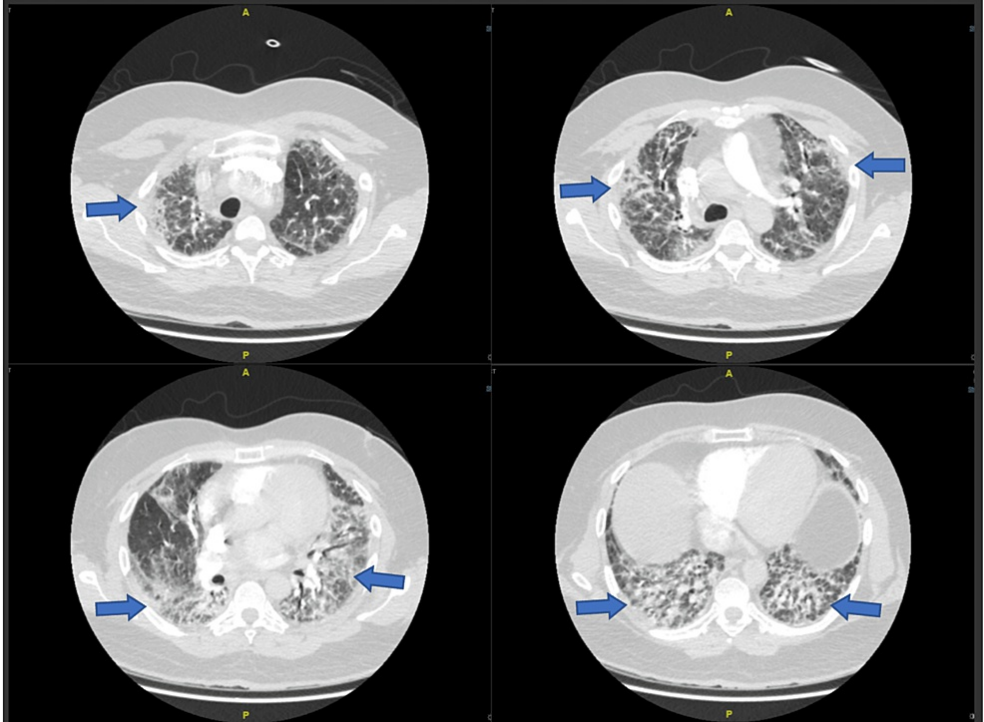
Patient’s chest computed tomography showing fibrotic nonspecific interstitial pneumonia pattern
